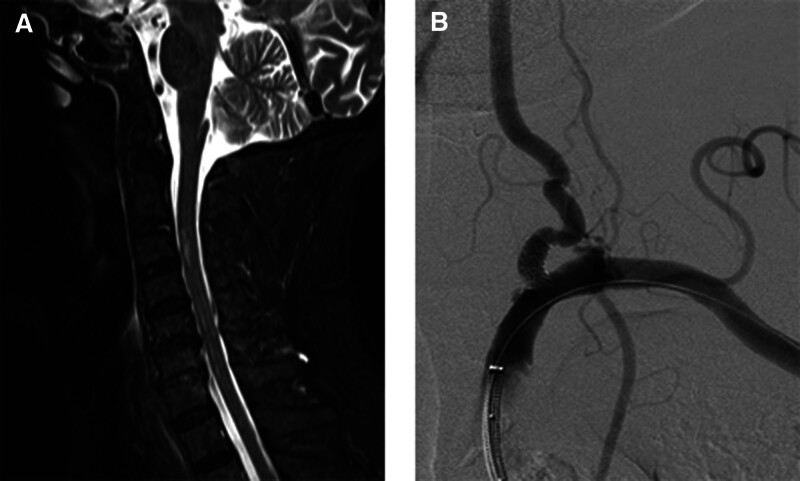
(A, B) 10 to 14 02:01 After continuing the communication with the family members, the emergency department underwent a DT examination of the head and MRI of the cervical vertebra again. A new acute cerebral infarction of the right cerebellum was considered after a radiographic review by a radiologist. T3 cervical infarction?/Cervical inflammation?
